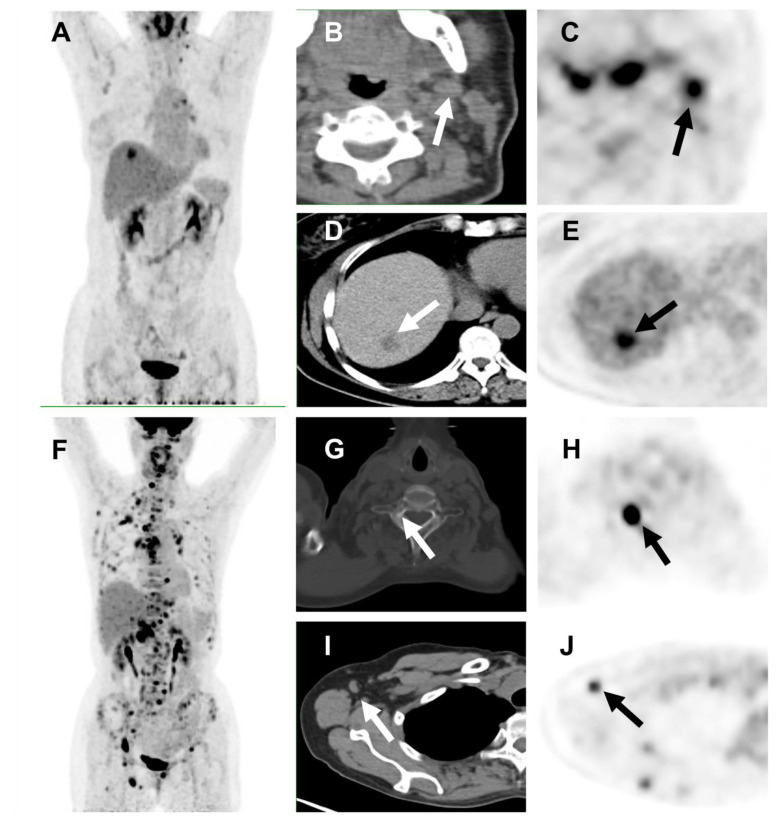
Representative cases of tumoral heterogeneity on ^18^F-FDG PET/CT and response to pyrotinib. (A–E) A 56-year-old woman underwent ^18^F-FDG PET/CT ((A), maximum intensity projection [MIP] image). The left cervical lymph node lesion had the highest uptake (B), CT image; (C), PET image; SUVmax = 7.94, SUVmean = 5.09), whereas the liver lesion had the lowest uptake ((D), CT; (E), PET, SUVmax = 6.94, SUVmean = 3.84). This patient’s median HI-intra was 1.68, and her HI-inter was 1.14. She has received pyrotinib treatment for 39.7 months and has not yet experienced tumor progression. (F–J) A 61-year-old woman who underwent ^18^F-FDG PET/CT ((F), MIP) showed multiple bone and lymph nodes metastases, with the highest uptake in the cervical vertebrae ((G), CT; (H), PET image; SUVmax = 21.72, SUVmean = 13.16) and the lowest uptake in the left axillary lymph node ((I), CT; (J), PET; SUVmax = 4.73, SUVmean = 3.19). This patient’s median HI-intra was 1.70, and her HI-inter was 4.59. She experienced disease progression after 3.6 months of pyrotinib treatment. Abbreviations: FDG, fluorodeoxyglucose; PET/CT, positron emission tomography/computed tomography; SUVmax, maximum standardized uptake value; SUVmean, mean standardized uptake value; HI-intra, intratumoral heterogeneity index; HI-inter, intertumoral heterogeneity index.
